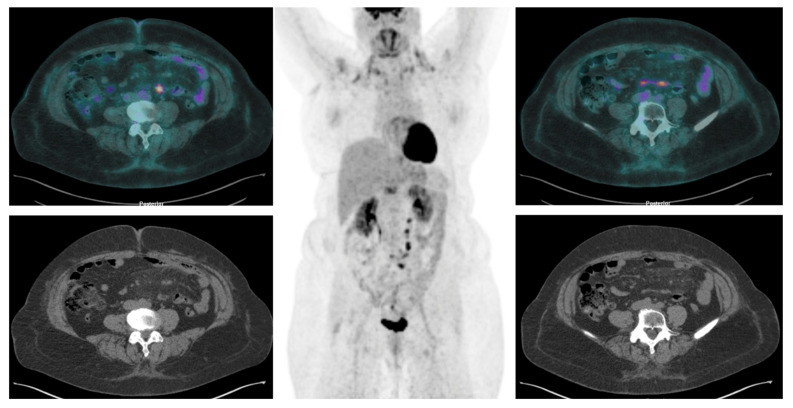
[^18^F]FDG PET/CT revealed a nodular hypermetabolic lesion in the mesenteric region and other foci of increased [^18^F]FDG accumulation in mesenterial soft tissue.
